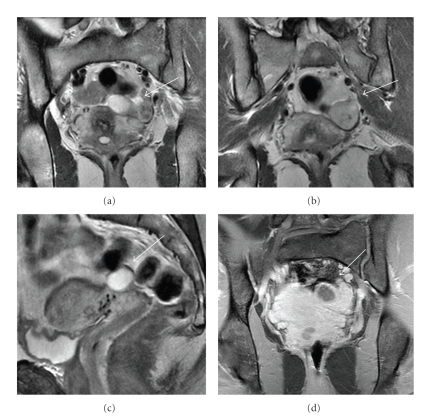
MRI of the pelvis (10/29/2007). Coronal T2-weighted images (a) and (b) demonstrate a left adnexal mixed solid and cystic mass (white arrow). The hyperintense T2 area represents the cystic component, and the isointense T2 area is the soft tissue component of the mass. Sagittal T2-weighted image (c) demonstrates the cystic component of the mass (white arrow). On coronal T1 Fat-Sat postcontrast image (d), the solid component of the mass enhances homogeneously after gadolinium administration; however, the cystic component is hypointense (white arrow.)
